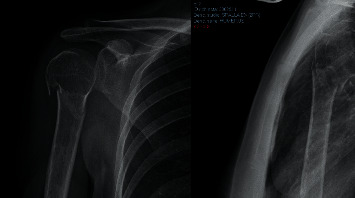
A standard anterior-posterior and axillary X-ray view of a PHF.
